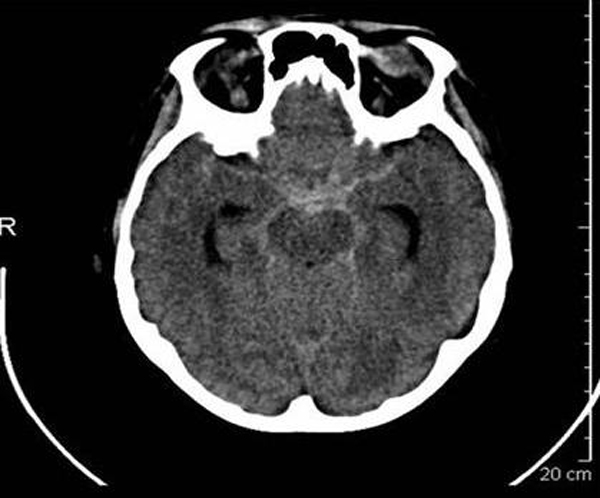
Noncontrast CT showing subarachnoid hemorrhage.
